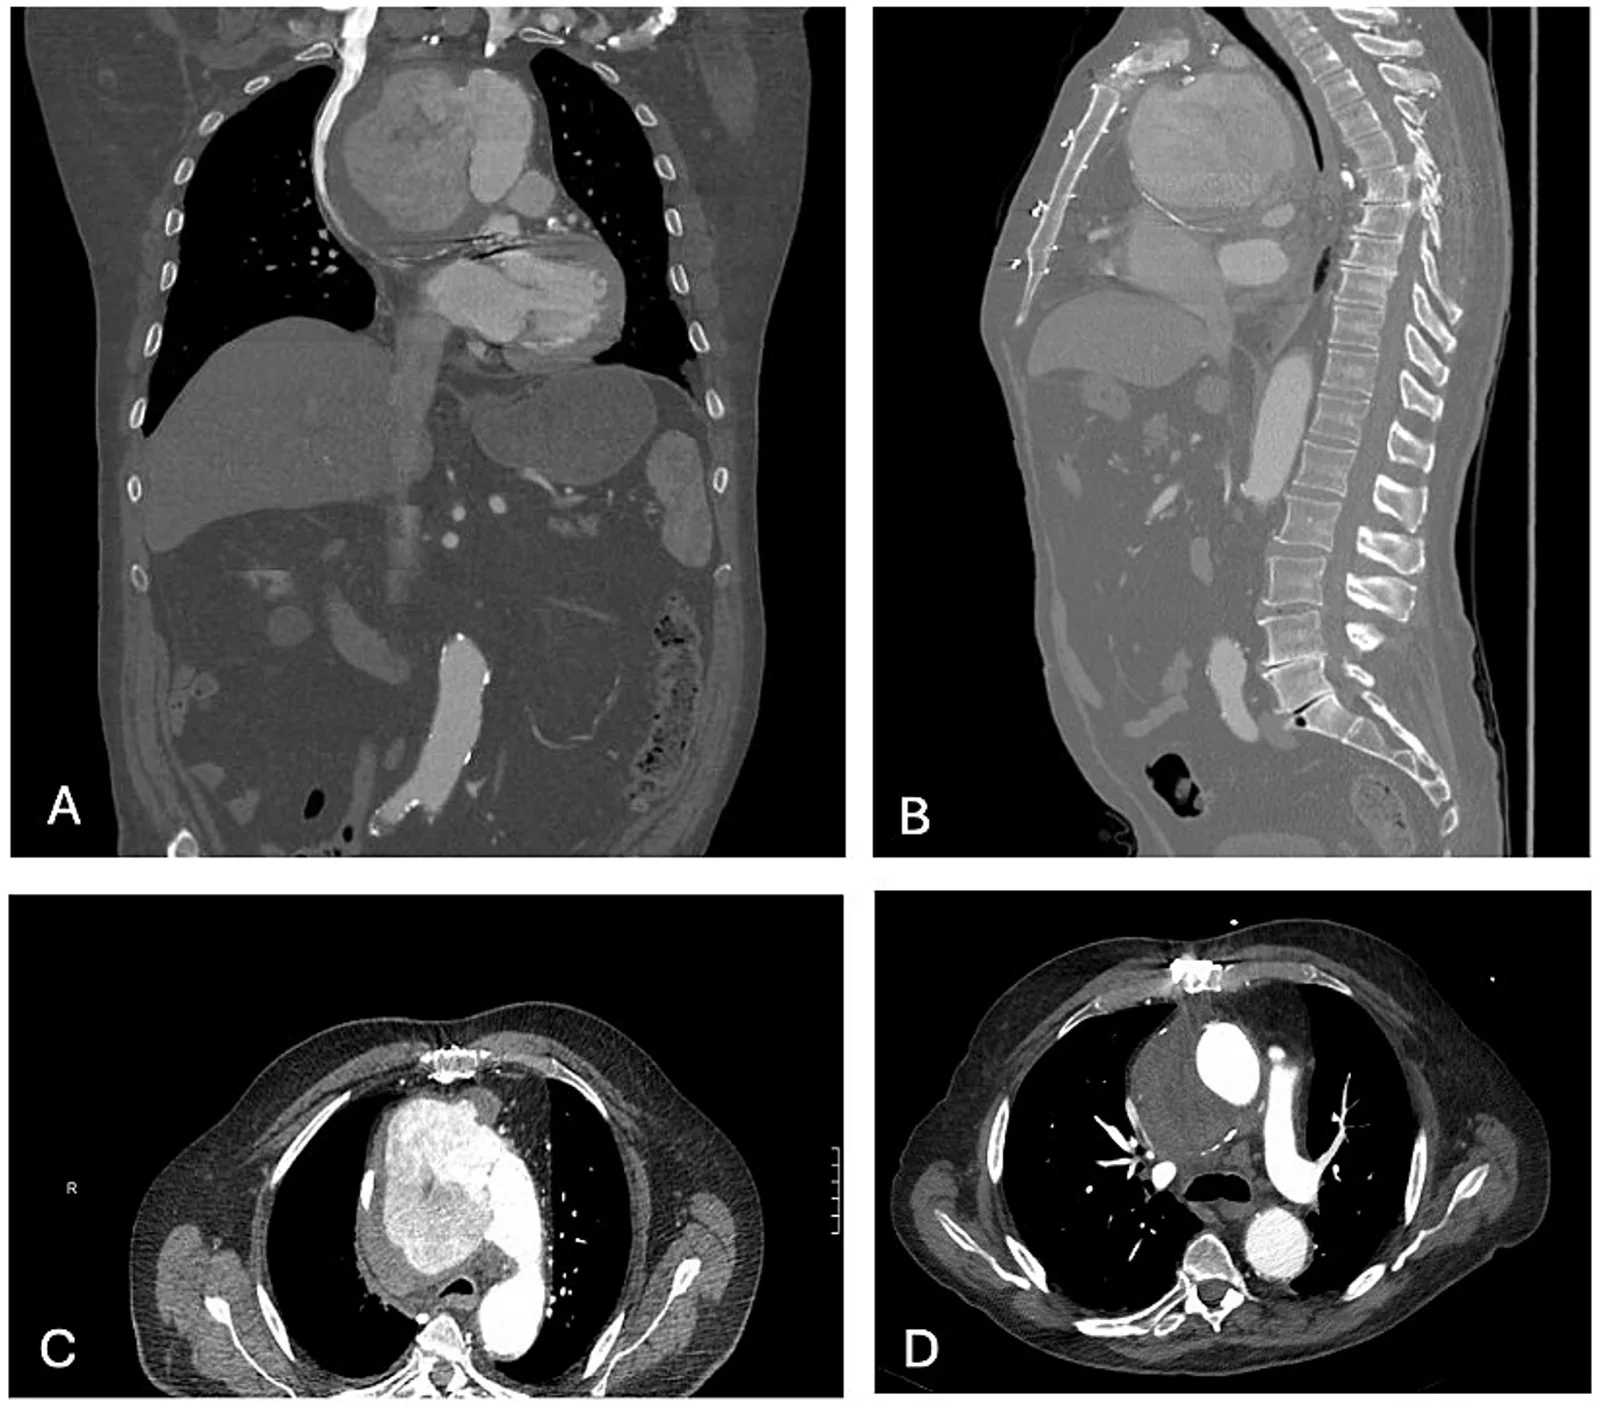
Preoperative CTA chest demonstrates massive pseudoaneurysm with compression of the superior vena cava, the main pulmonary artery and significant displacement of the innominate and left common carotid arteries. The wall of the pseudoaneurysm is adherent to the posterior sternal table (A-C). Postoperative CTA demonstrates the size of the ascending graft in comparison with the residual pseudoaneurysm cavity (D).
